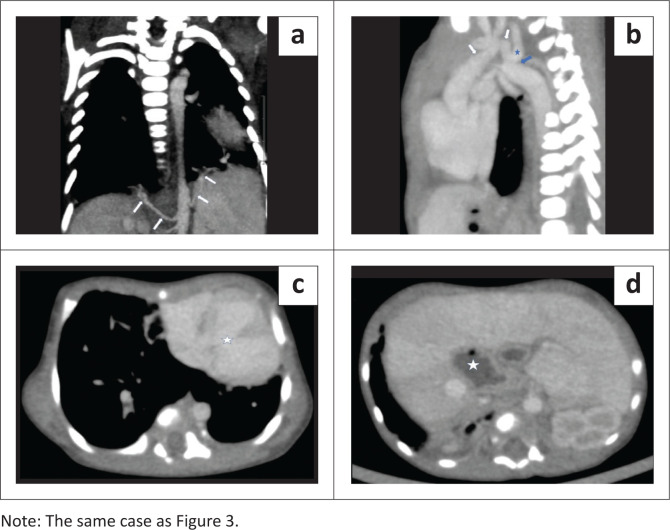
(a) Coronal oblique maximum intensity projection (MIP) image of the contrast enhanced computed tomography (CECT) chest demonstrates aberrant systemic arterial supply from the abdominal aorta to both lower lobes. (b) Sagittal oblique MIP image on mediastinal window shows a hypoplastic aortic arch (white arrows) with distinct narrowing of the aortic isthmus (blue arrow), just distal to the origin of the left subclavian artery (blue star), representing pre-ductal coarctation of the aorta. (c) Axial CECT image demonstrates a large ventricular septal defect (star). (d) Axial CT through the upper abdomen shows the midline position of the stomach (solid star), an absent spleen and a relatively large, transversely placed liver, suggesting situs ambiguous with right isomerism.
